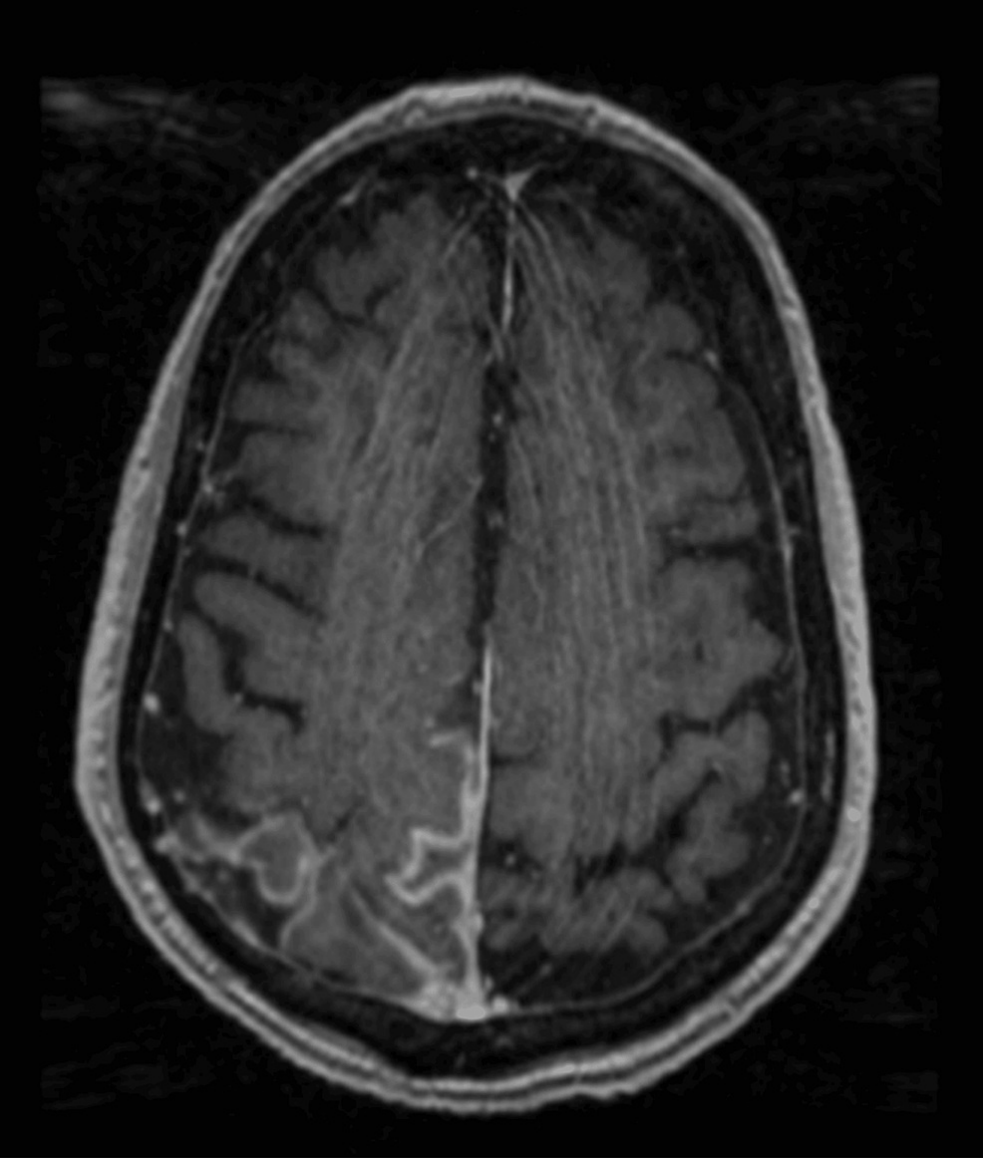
Initial MRI with contrast, fluid-attenuated inversion recovery image; leptomeningeal enhancement in the right parasagittal region MRI: magnetic resonance imaging
